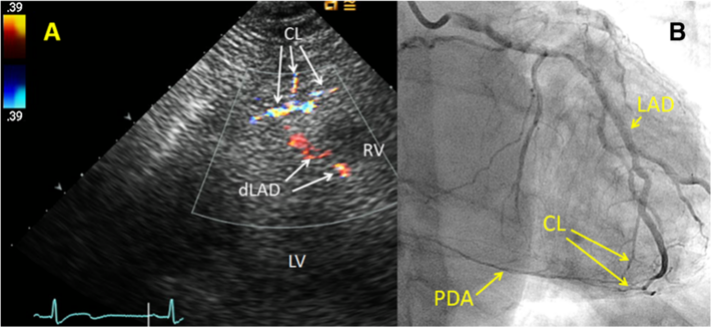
Example of transthoracic Doppler findings of collateral blood flow in the apical region, compared with coronary angiography. (A) In a modified parasternal long-axis view focusing on the apex and distal anterior interventricular sulcus, several epicardial and intramyocardial collaterals (CL) originates from the distal left anterior descending coronary artery (dLAD). (B) Angiogram of the left coronary artery demonstrates apically located epicardial and intramyocardial collateral (CL) blood supply from the dLAD to the posterior descending coronary artery (PDA), which shows retrograde flow. Angiogram of the right coronary artery demonstrated a proximal PDA occlusion (not shown). LAD = left anterior descending coronary artery, LV = left ventricle, RV = right ventricle.
